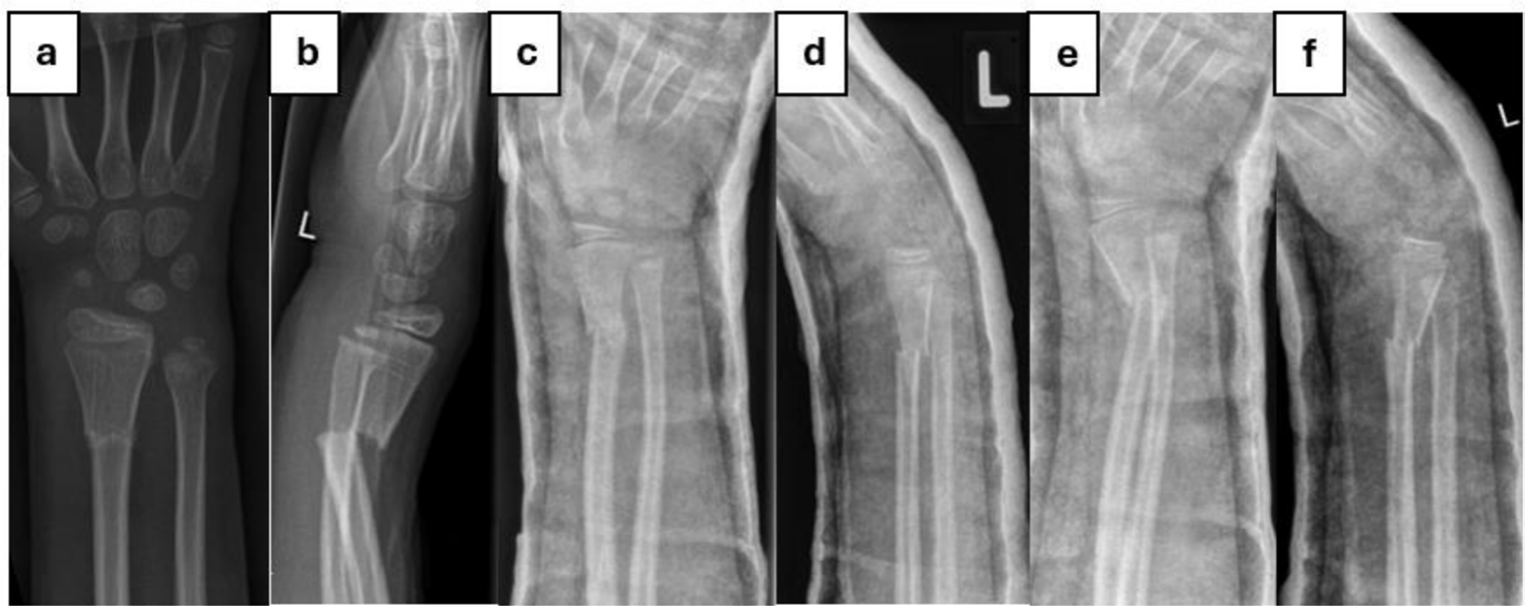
a – b, anterior-posterior and lateral radiographs of a dislocated diametaphyseal radius fracture in a 6-year-old boy at the day of the accident (day 0). The forearm fracture index is 1.2, the angulation in the lateral radiograph is 30° and the angle of the fracture line in the a.p. radiograph is approximately 2°. Hence, the risk for secondary dislocation according to the proposed risk prediction score in Table 3 is 13.2%. Initially, the fracture was managed conservatively; c – d, radiologic imaging shows an acceptable retention of the reduced fracture on day 7; e – f, radiologic imaging shows a considerable secondary dislocation of the fracture, particularly in the anterior-posterior plane on day 16
